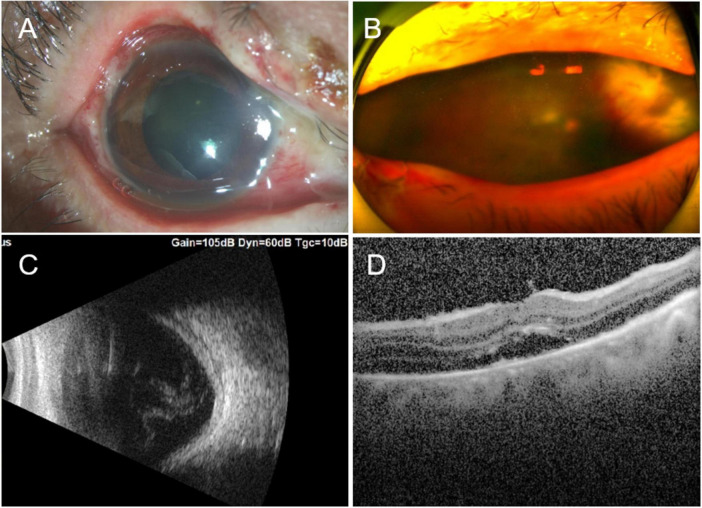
(A) Two weeks after the ocular burn, edematous eyelids, nasal limbal ischemia (Roper-Hall category II), mixed congestion of the conjunctiva, and corneal edema were observed, together with panel (B) vitreous opacity (3+) and a large yellow–white lesion on the nasal peripheral retina, which resembled a subretinal abscess. (C) B-scan ultrasound showed ocular edema and vitreous opacity. (D) OCT showed subretinal fluid in the macula. OCT, optical coherence tomography.
